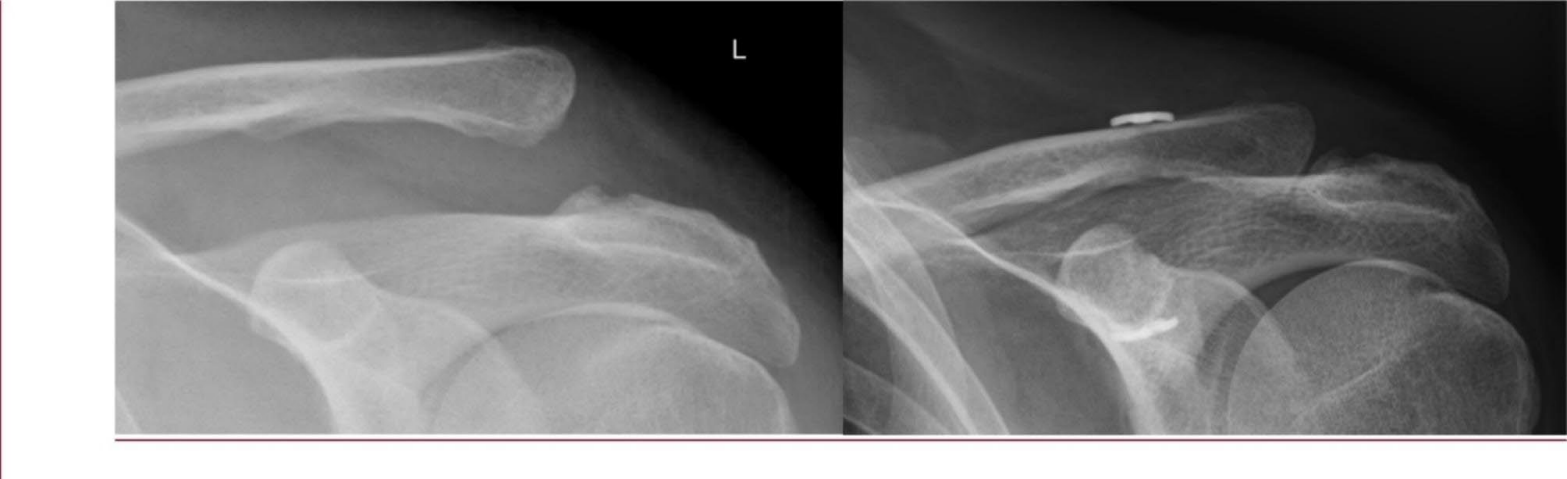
X-rays preoperatively/ like conservativly (left) and 6 Weeks postoperatively with high satisfaction
